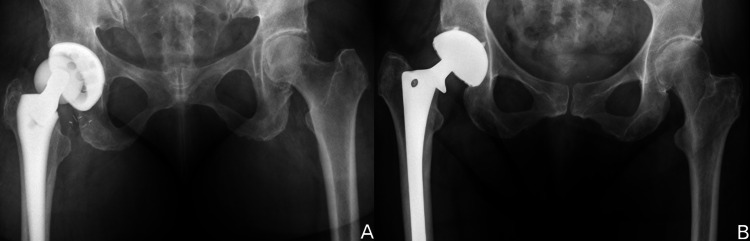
Patients with recurrent instability related to implant malposition. (A) AP radiograph of a vertically malpositioned cup. (B) AP radiograph of a retroverted cup with asymmetric bearing wear.
